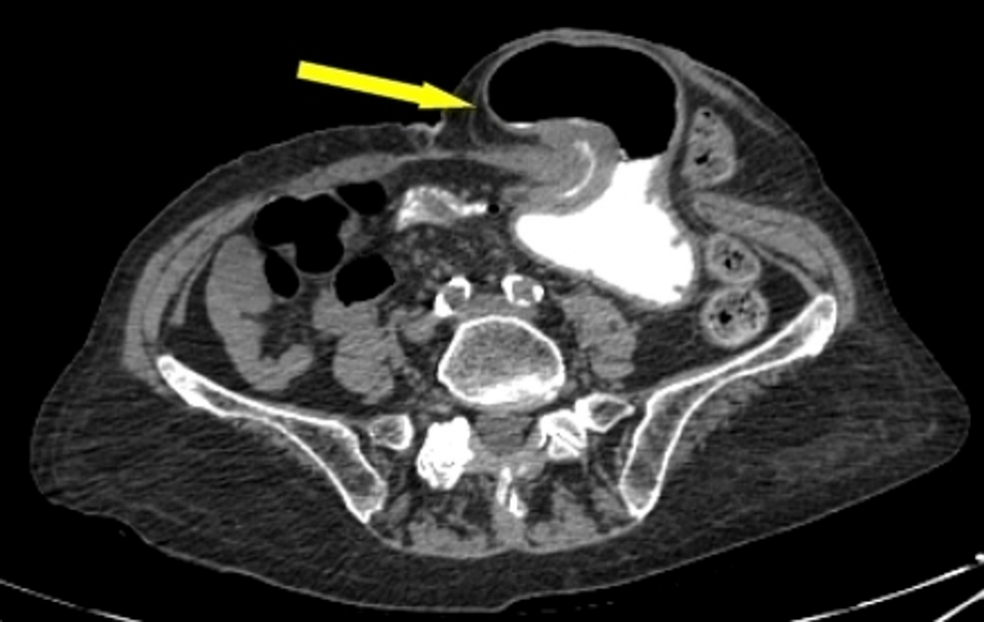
Axial view of abdominal CT scan, yellow arrow indicates the presence of stomach into parastomal hernia.
